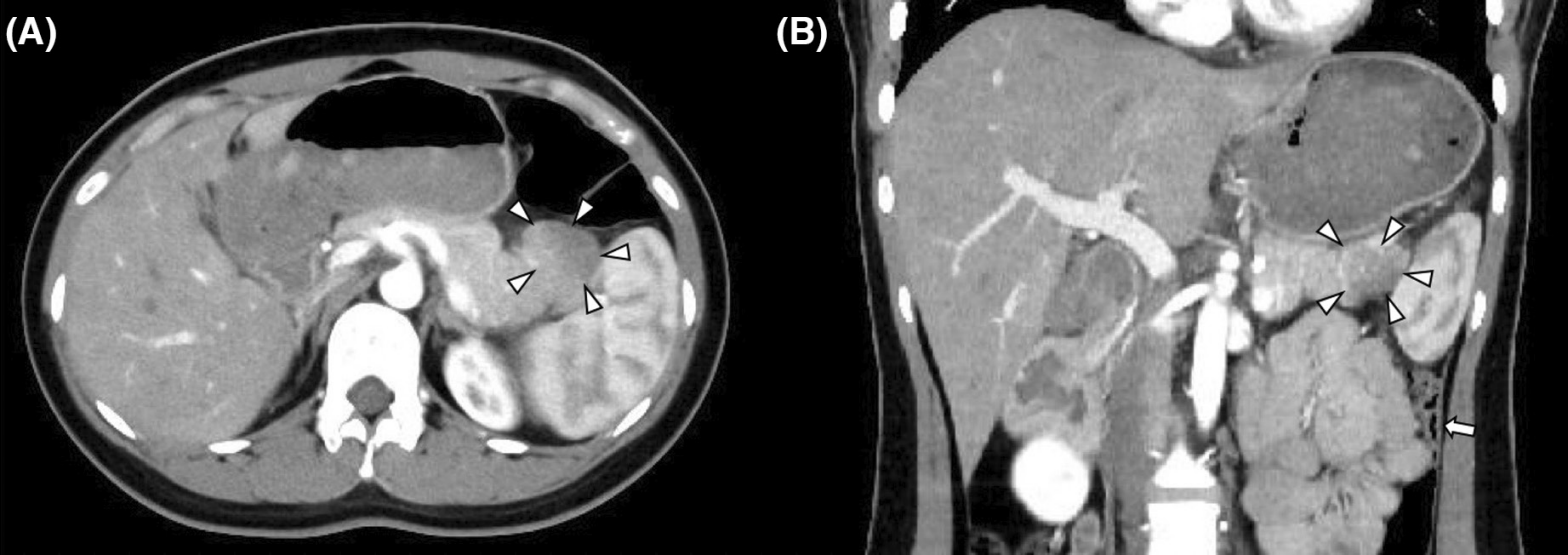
Abdominal intravenous contrast‐enhanced CT. (A) Axial section (B) Coronal section. Poor contrast area in the tail of the pancreas (arrowhead). Narrowing of the descending colon (arrow).
